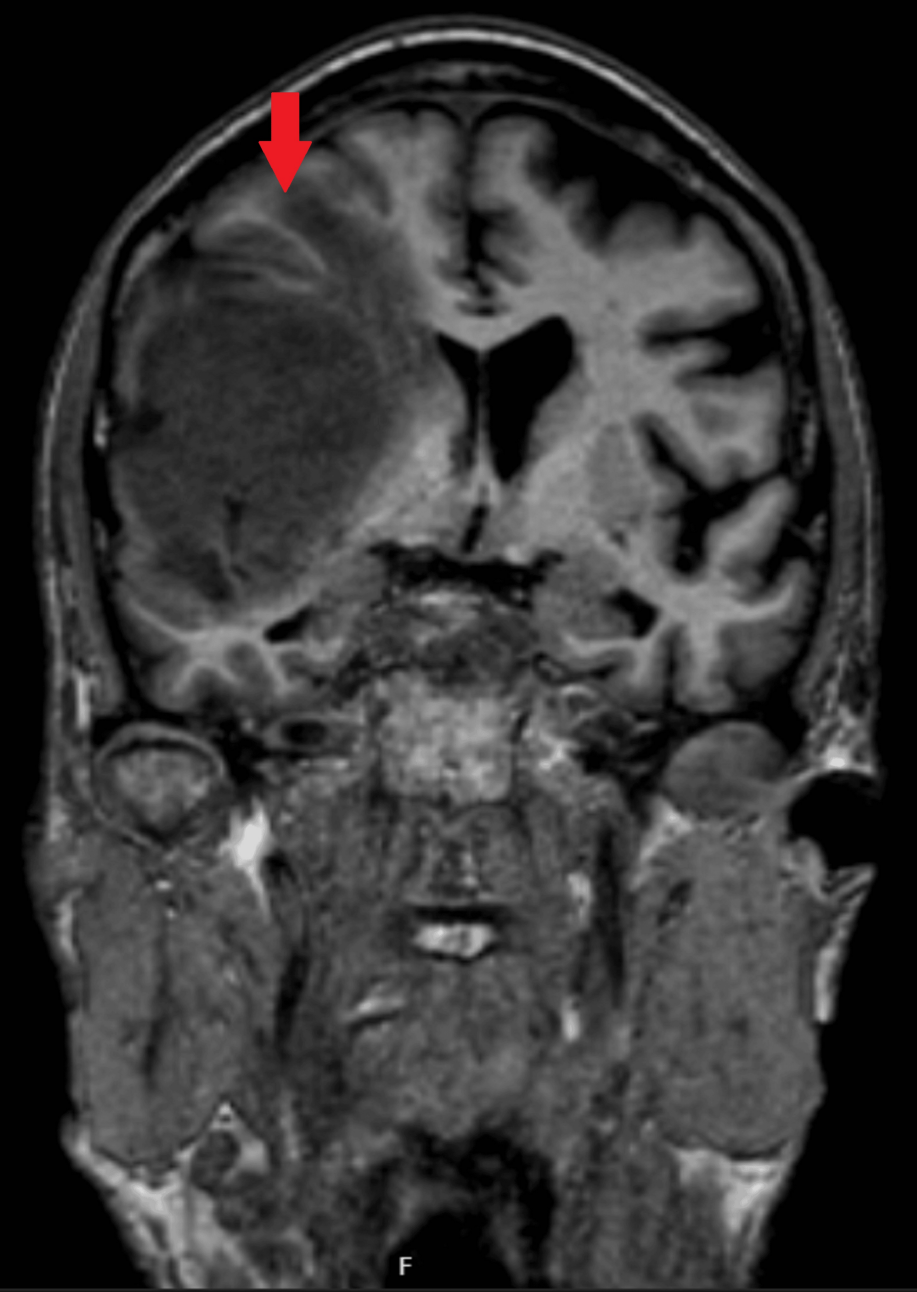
Coronal section of MRI shows a brain mass
